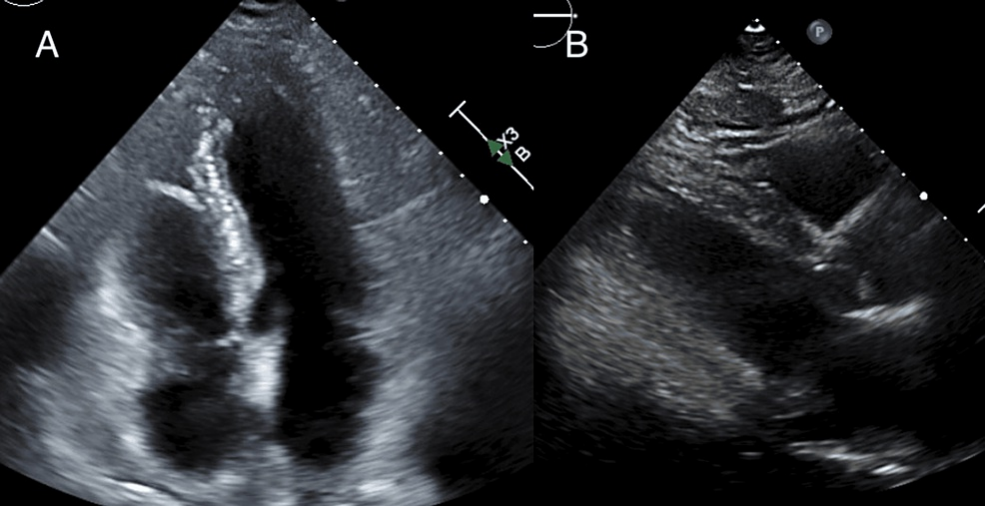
Echocardiogram images The ventricular and septal wall thickness appeared to be normal on the apical four-chamber view (A) and parasternal long-axis view (B).
